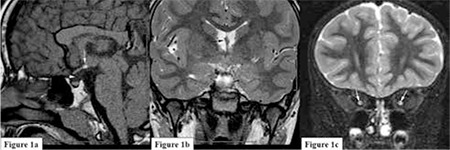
In the T1-weighted sagittal section; a) ectopic neuro-hypophysis (white arrow) is present in the hypothalamic region outside sella turcica. In the middle line, no hypophyseal infundibulum (arrow head) is present. In the T2-weighted coronal section; b) septum pellucidum is present in the middle line (arrow). In the coronal section fat-suppressed; c) hypoplastic bilateral optic nerves are demonstrated (arrows)
